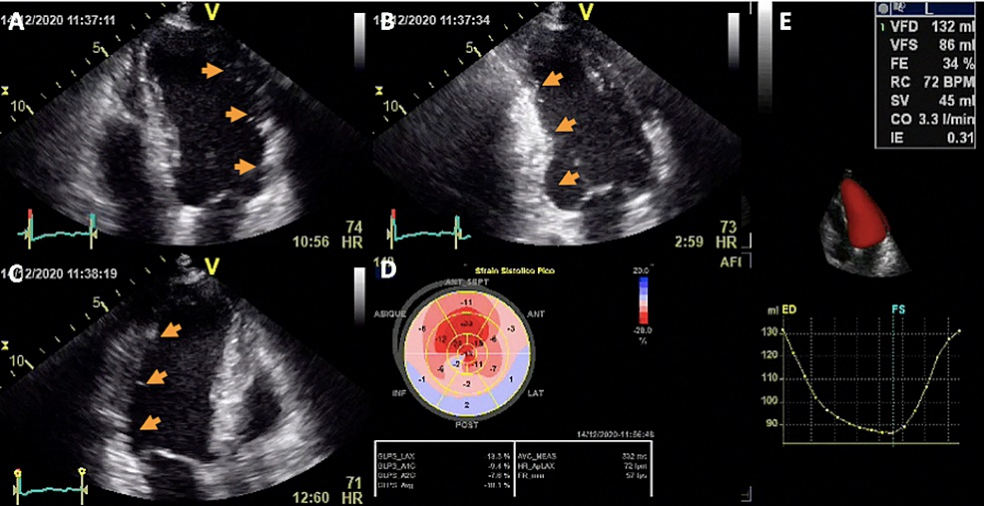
Transthoracic echocardiography findings of the patient Two-dimensional left ventricular apical views at the A: four-chamber view, B: two-chamber view, and C: three-chamber view. D: bullseye map of left ventricular global longitudinal strain. E: three-dimensional echocardiography evaluation of left ventricular volumes and ejection fraction
